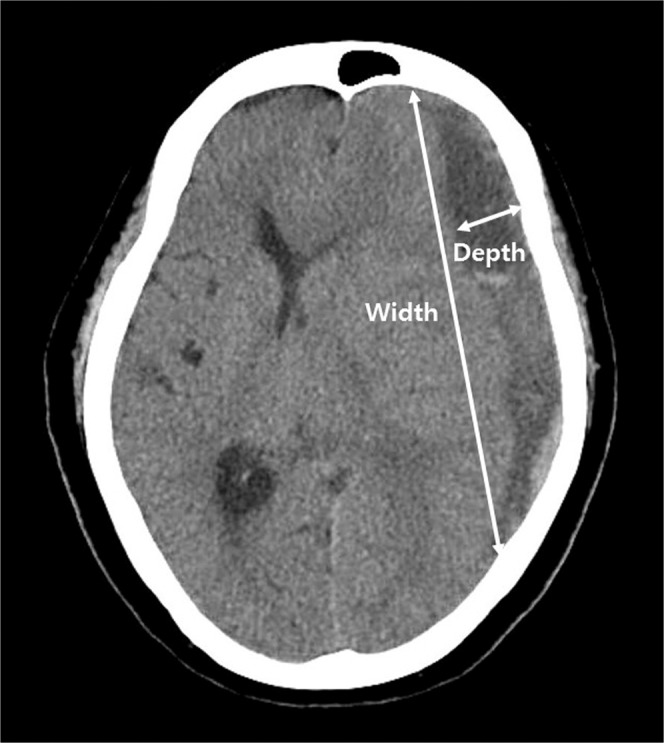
Computed tomography (CT) scan demonstrating measurements of width and depth for subdural hematoma.
